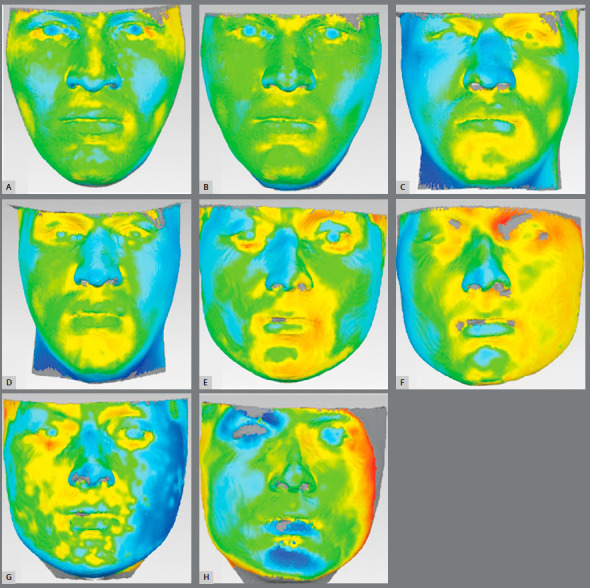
Color map models with the best and worst results within the sample: A) best AFL x CBCT; B) best AAL x CBCT; C) best ZFL x CBCT; D) best ZAL x CBCT; E) worst AFL x CBCT; F) worst AAL x CBCT; G) worst ZFL x CBCT;H) worst ZAL x CBCT.
